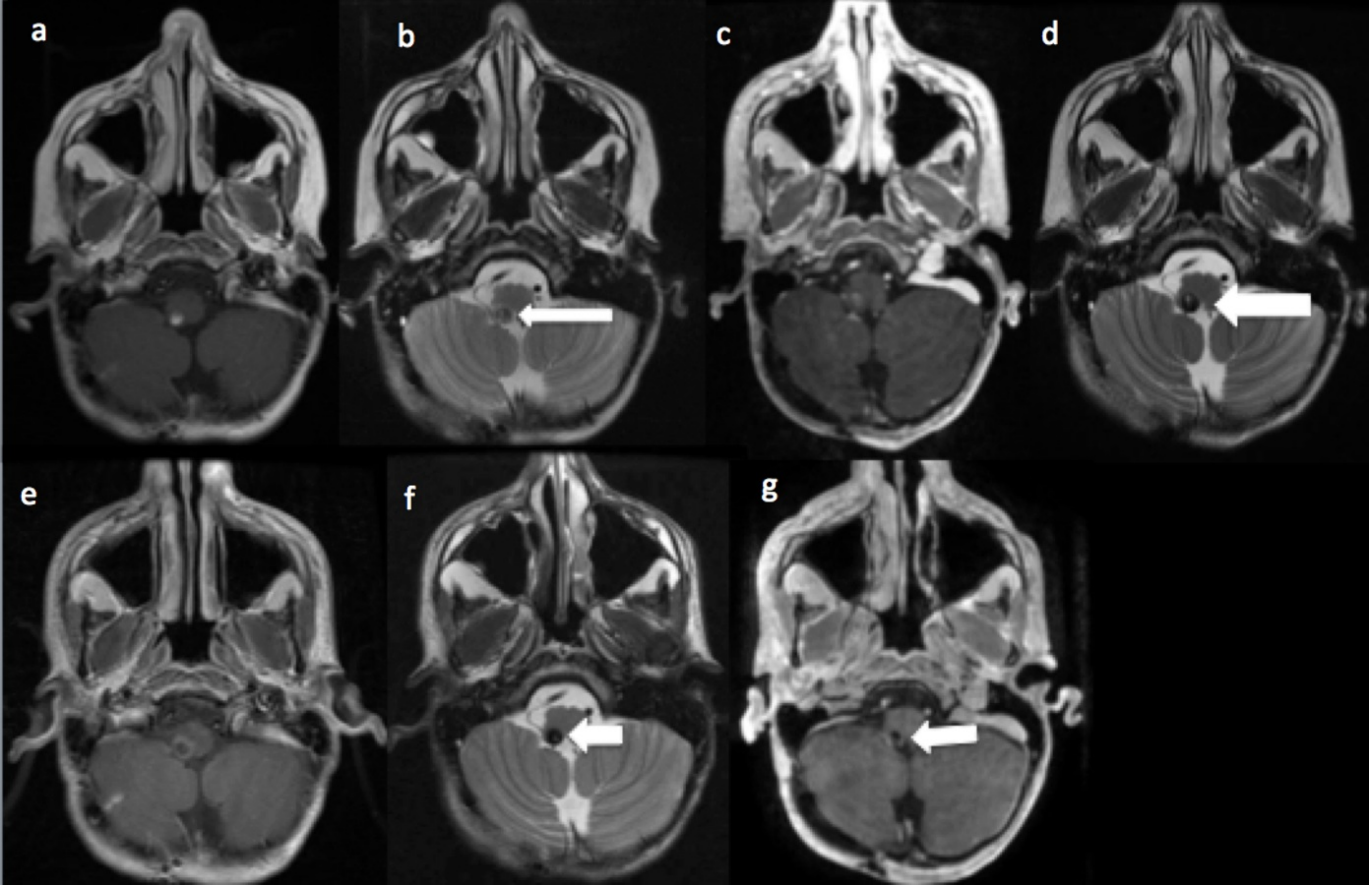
Right medullary cavernoma initially diagnosed on routine follow-up imaging seven years after the initial resection of the primitive neuroectodermal tumor (PNET) Right medullary cavernoma size over time. (a/b): Axial T1 and T2 images depicting first radiographic evidence of the right medullary cavernoma (white arrow), with some T1 with contrast enhancement seven years after initial surgery/radiation for melanotic PNET. (c/d): Axial T1 and T2 images depicting one-year follow-up study after initial cavernoma findings demonstrating less T1 with contrast enhancement, and small increase in size on T2 image (white arrow). (e/f): T1 and T2 images depicting cavernoma six weeks prior to presentation with intractable hiccups. The pattern of enhancement on T1 image is now ring like, but the overall size on T2 image is slightly smaller. (g): Post-resection T1 with contrast revealing complete resection of previous cavernoma (white arrow).
